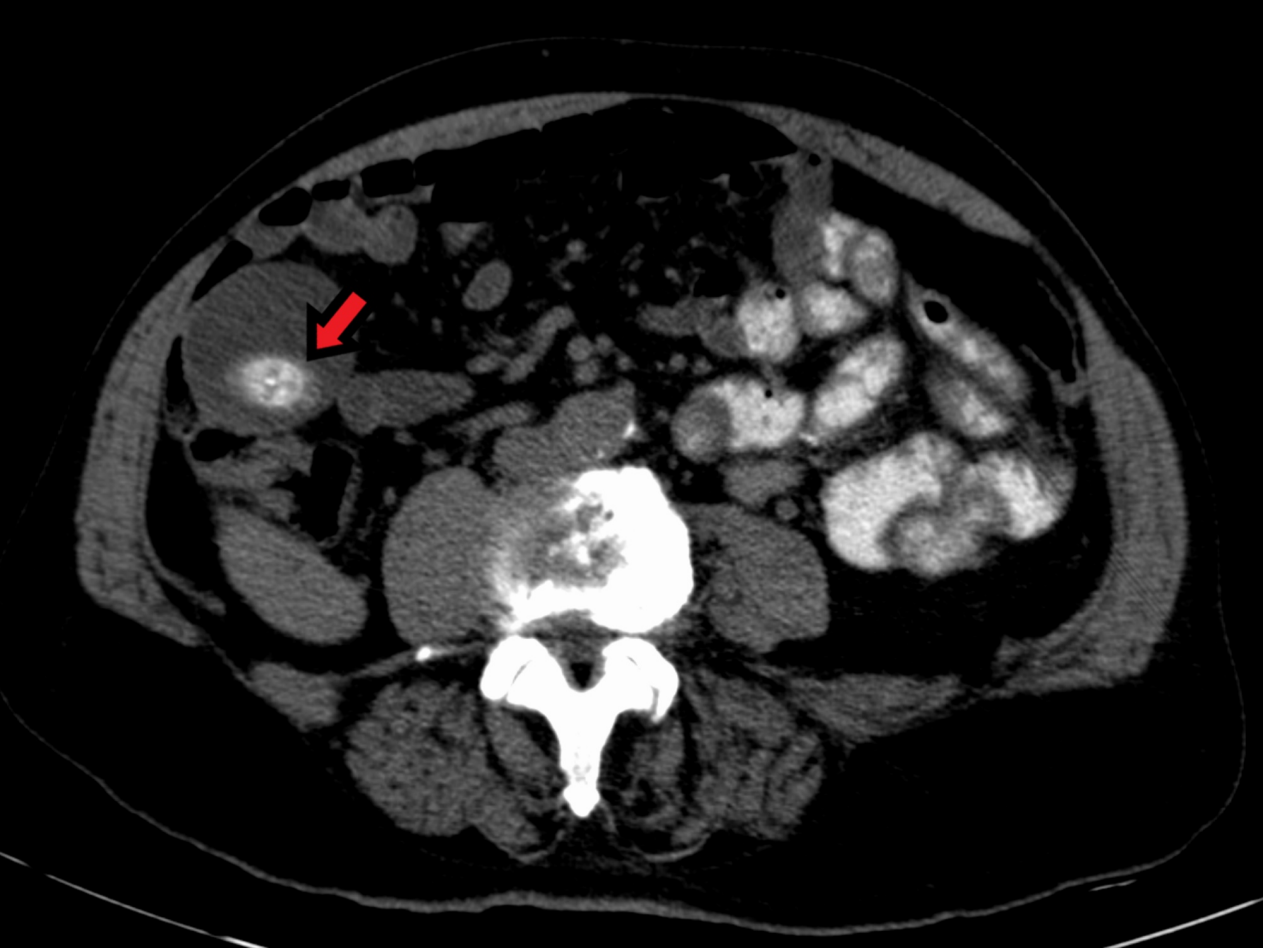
Axial view CT abdomen with oral contrast demonstrating a significantly dilated gallbladder. A 3 cm calcification is seen in the dependent portion of the fundus (arrow) CT - Computed tomography
